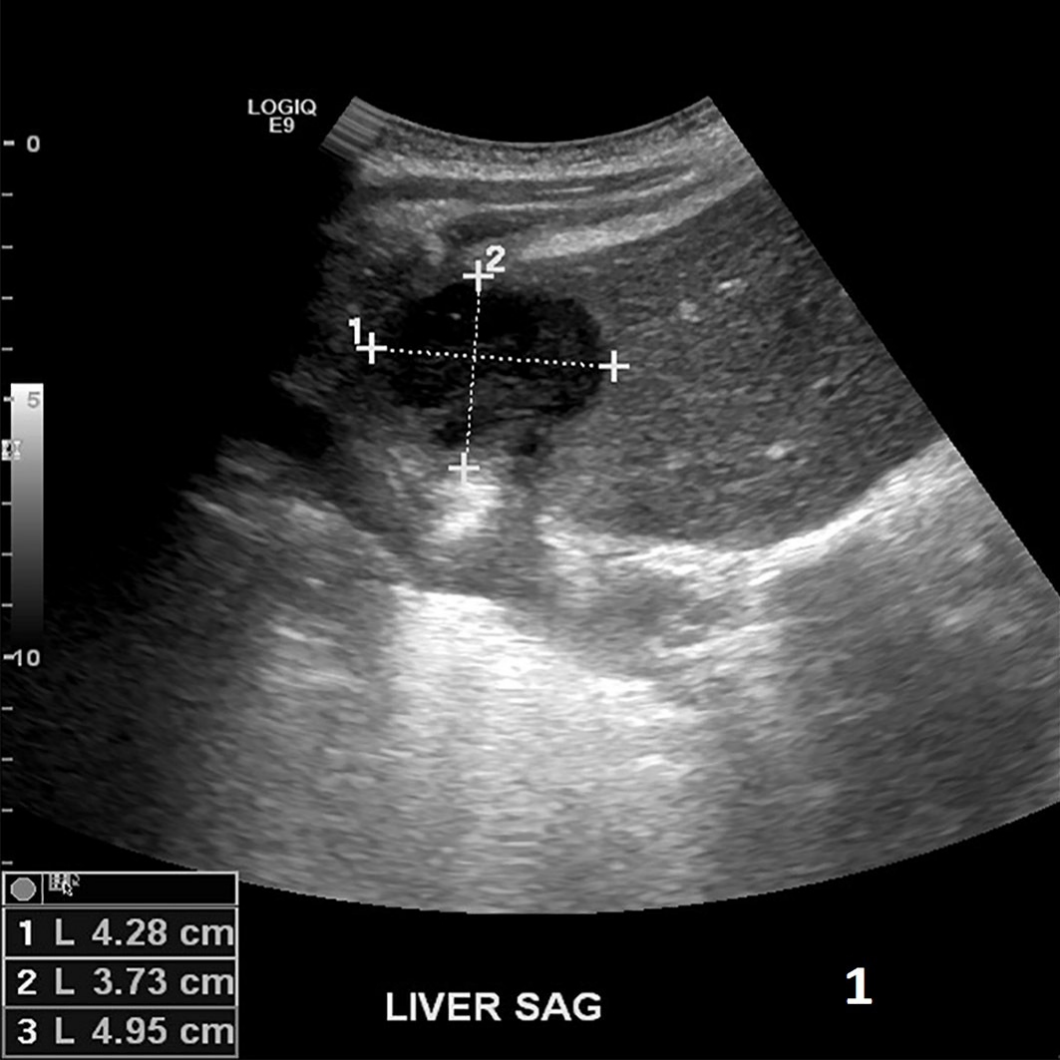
Right upper quadrant sonogram showed 4.1x0.9x2.7 cm cystic formation/well-defined fluid collection in the right upper abdomen anterior to the right liver lobe
